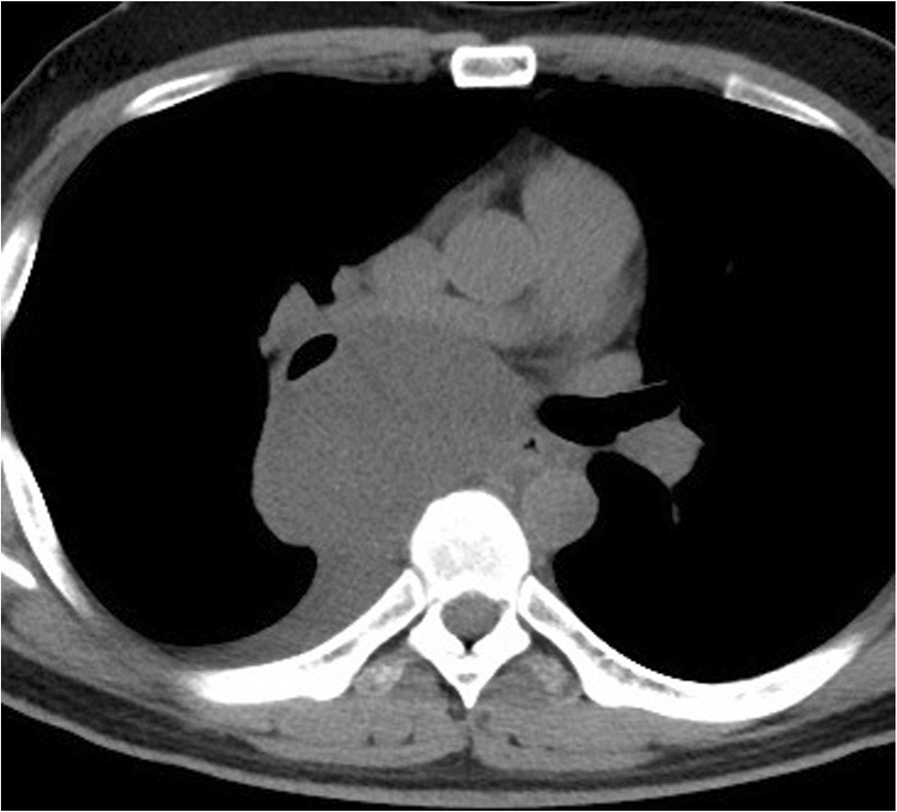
Initial chest computed tomography scan showing the mediastinal tumor measuring approximately 60 mm in length with right pleural effusion
